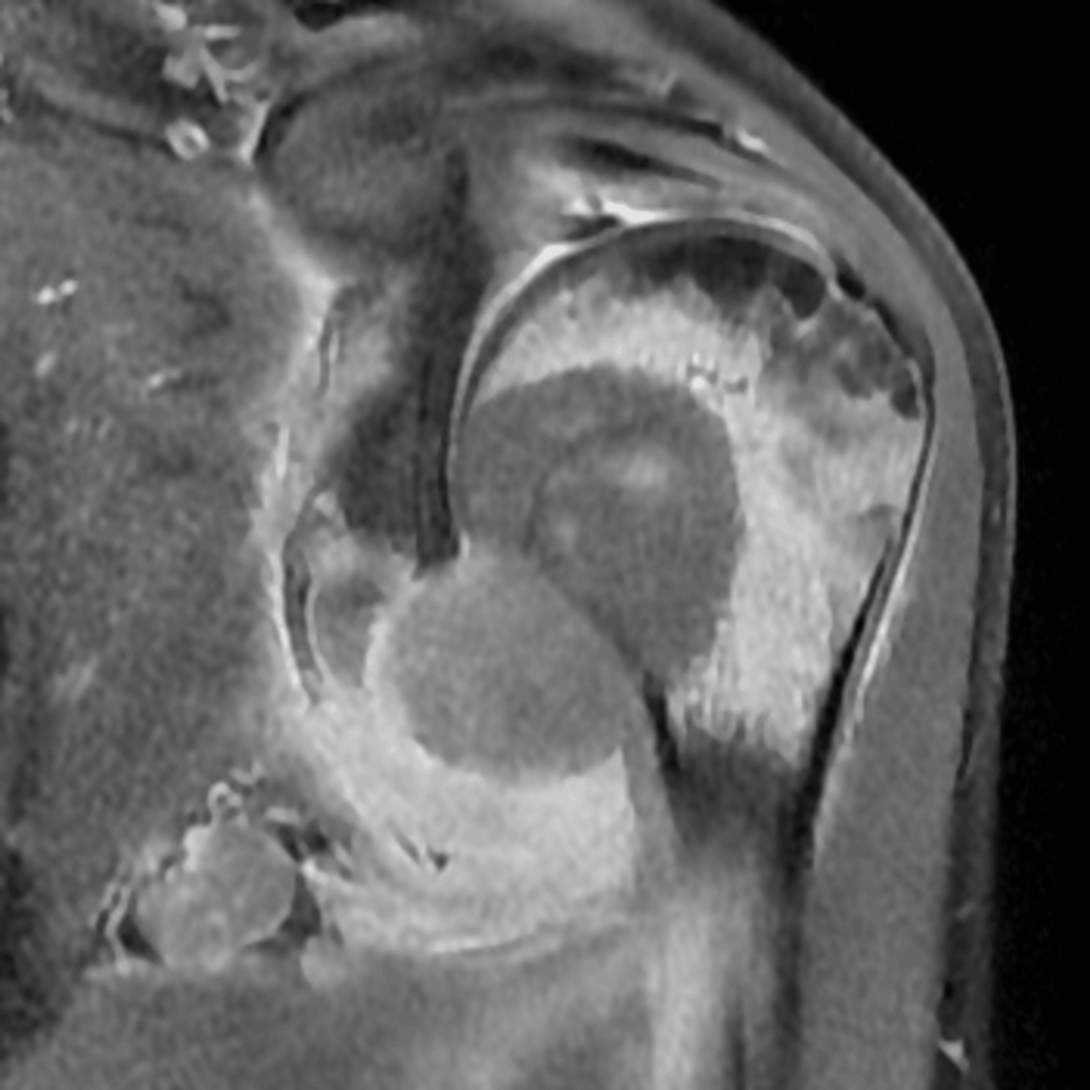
Post-contrast coronal T1-weighted fat saturated coronal image Mild uniform post-contrast enhancement of the mass is demonstrated.
